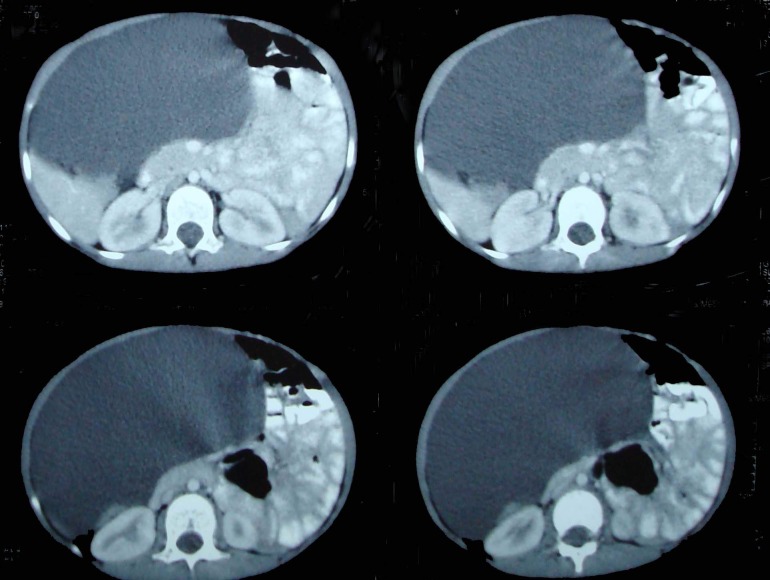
Figure 2: CT scan showing position of the cyst just beneath the anterior abdominal wall with compression of the adjacent viscera.
